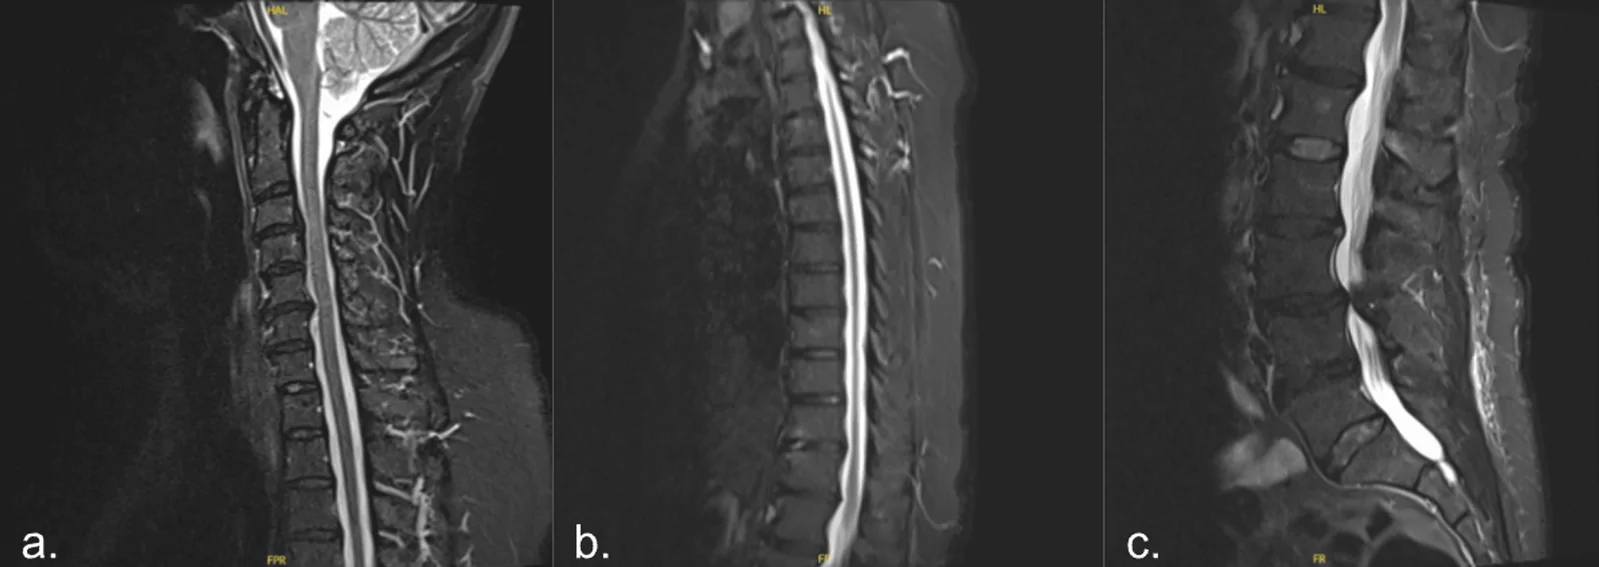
Sagittal short tau inversion recovery (STIR) MRI of the cervical (a), thoracic (b), and lumbar (c) spine demonstrating moderate-to-severe multilevel degenerative changes throughout the spine, including severe L3–L4 spinal canal stenosis and grade 1 L4–L5 spondylolisthesis.
